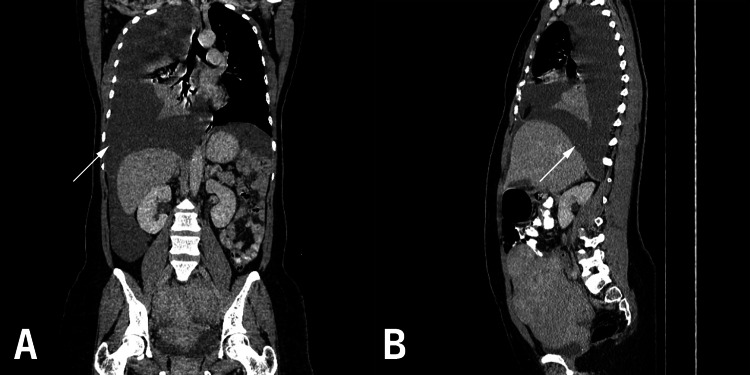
A: CT with contrast (coronal view) showing the pleural Effusion and consolidation in the adjacent lung parenchyma. Fig 5.B. CT with contrast (sagittal view) showing the pleural effusion.
